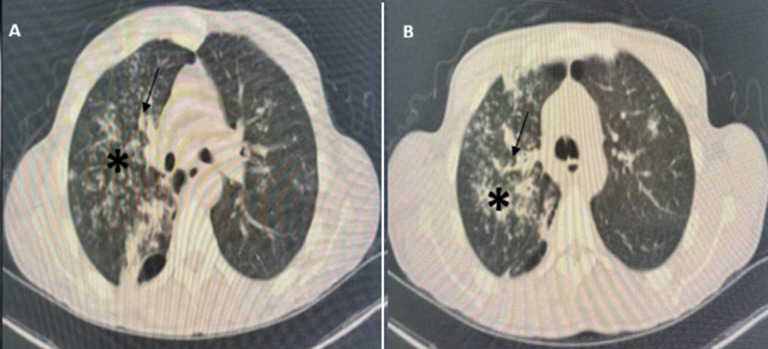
A,B) thoracic computed tomography (CT) of both lungs of the patient; consolidation with centrilobular branching micronodules, consistent with a tree-in-bud pattern, predominantly affecting the right upper lobe (*); peribronchovascular thickening (↓)
